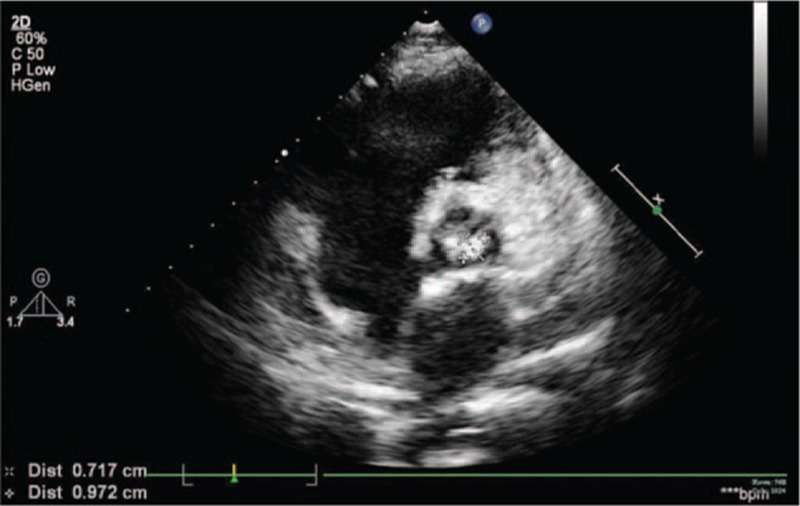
The echocardiogram showed a strong echo of 0.97∗0.72 cm at the valvula coronaria sinistra valvae aortae.
